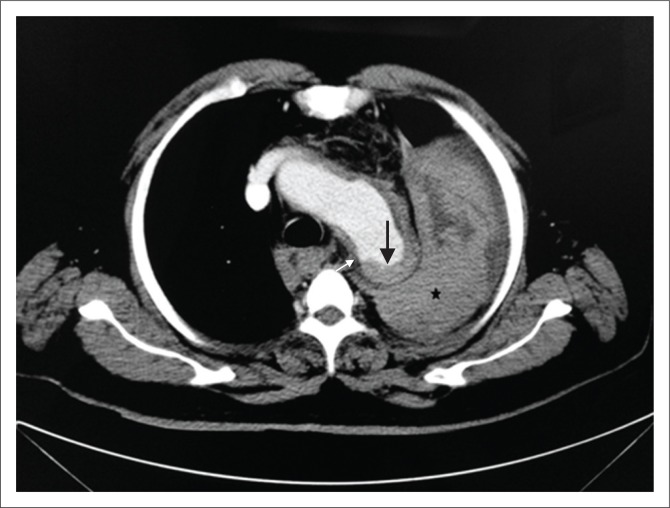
Ruptured penetrating atherosclerotic ulcer (PAU) with contained haematoma. Contrast-enhanced computed tomography scan of thorax showing a ruptured PAU along the posterior aortic wall (white arrow) with adjacent intramural haematoma (black arrow) appearing as hyperdense aortic wall thickening and a contained haematoma in left pleural space (*). Findings were confirmed at surgery.
